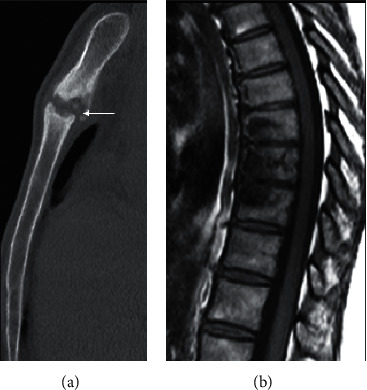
A 27-year-old female with SAPHO. There is hyperostosis and osteitis of the sternomanubrial junction on the sagittal CT (a). Contiguous involvement of multiple vertebral bodies anteriorly with sparing of the intervening disc space as demonstrated on sagittal T1 thoracic spine is typical of this condition (b).
